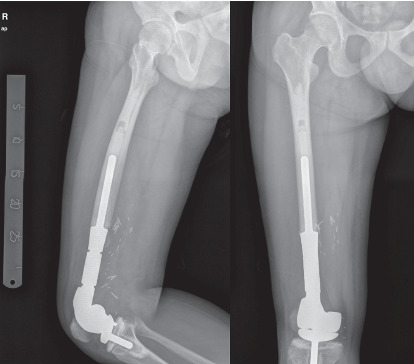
Postoperative radiographs of a 29-year-old male patient (case number 3) who underwent extra-articular resection and replacement with endoprosthesis.
